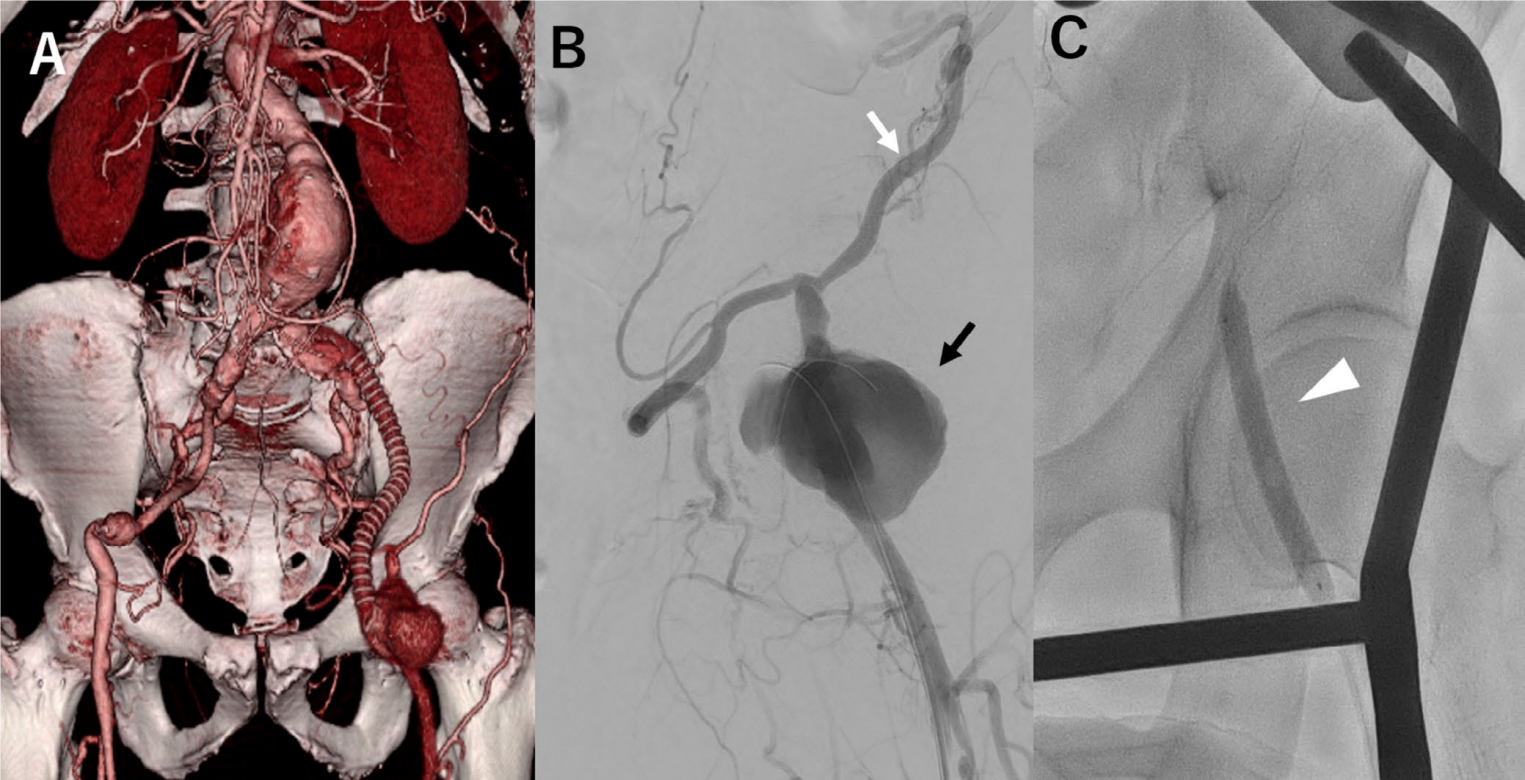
(A) Preoperative computed tomography showed abdominal aortic aneurysm and left femoral pseudo aneurysm. (B) Angiography revealed femoral pseudo aneurysm (black arrow) and circumflex iliac artery (white arrow). (C) Occlusion balloon was placed at common femoral artery (white arrow head).
